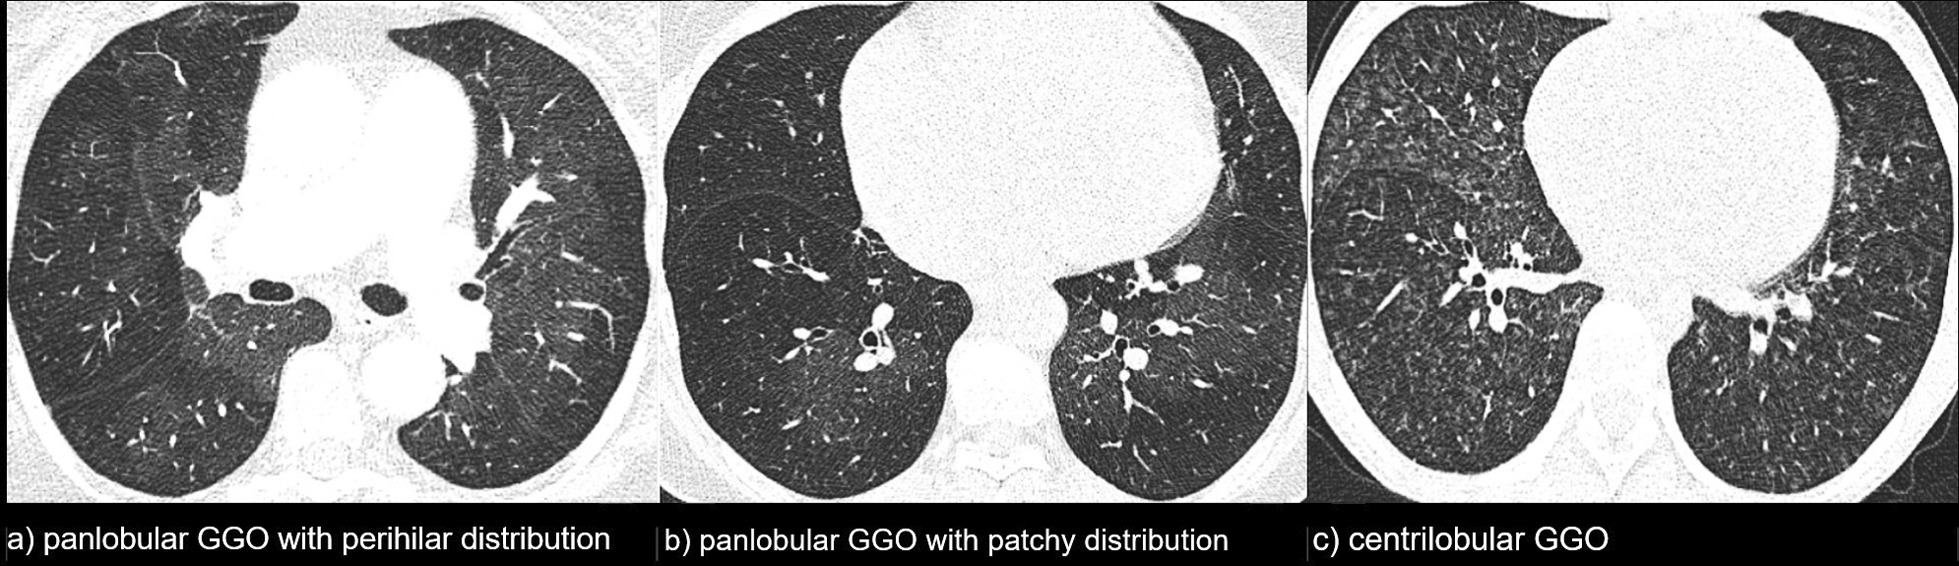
Chest HRCT scans of IPAH patients showing different patterns of ground-glass opacifications (GGO)
